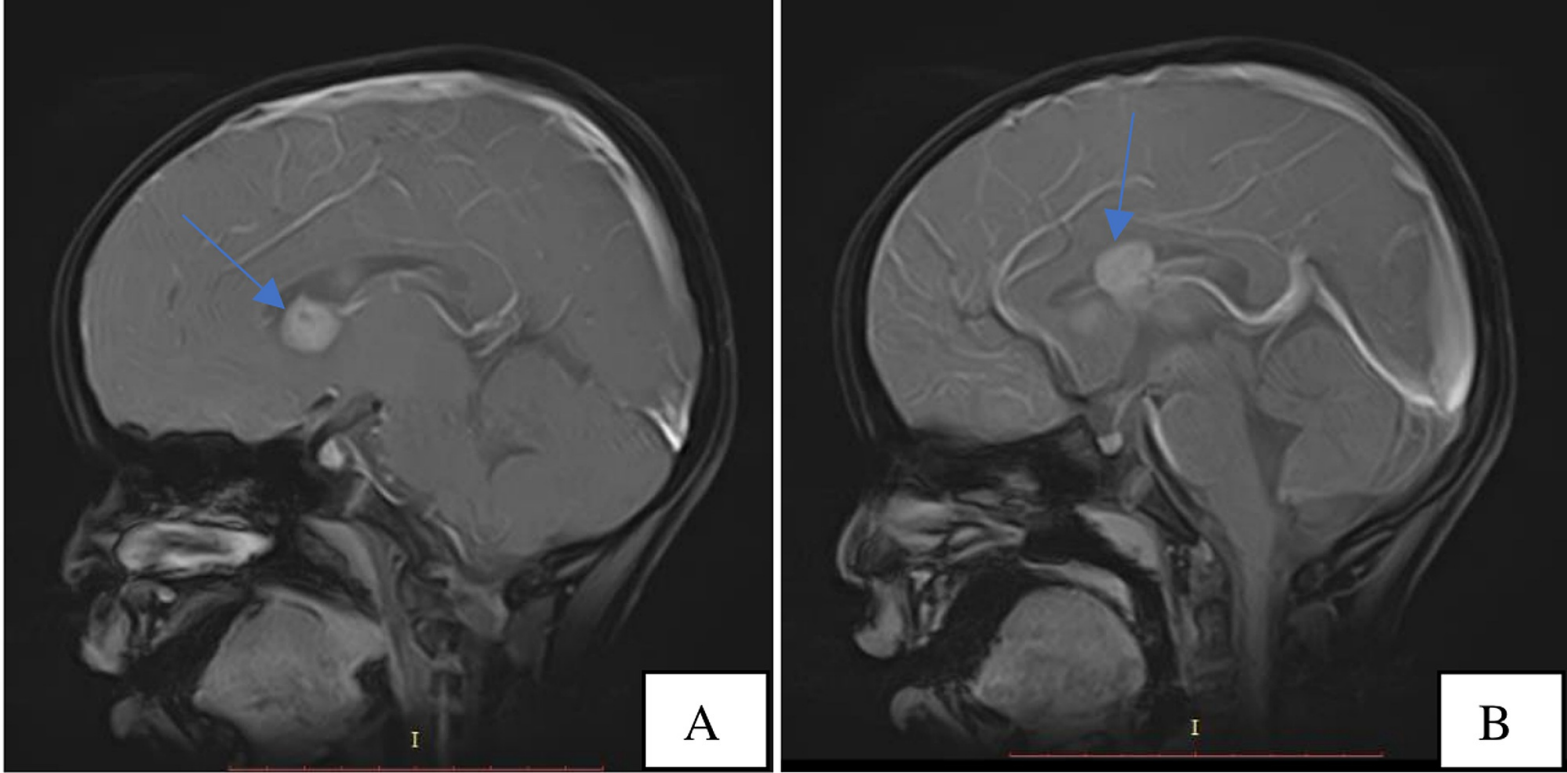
MRI, T1 Right para-sagittal (4A) and mid-sagittal FatSat contrast enhanced images (4B) showing the right & left Sub Ependymal Giant cell Astrocytomas approaching the Foramina of Monroe with no resultant hydrocephalus.
